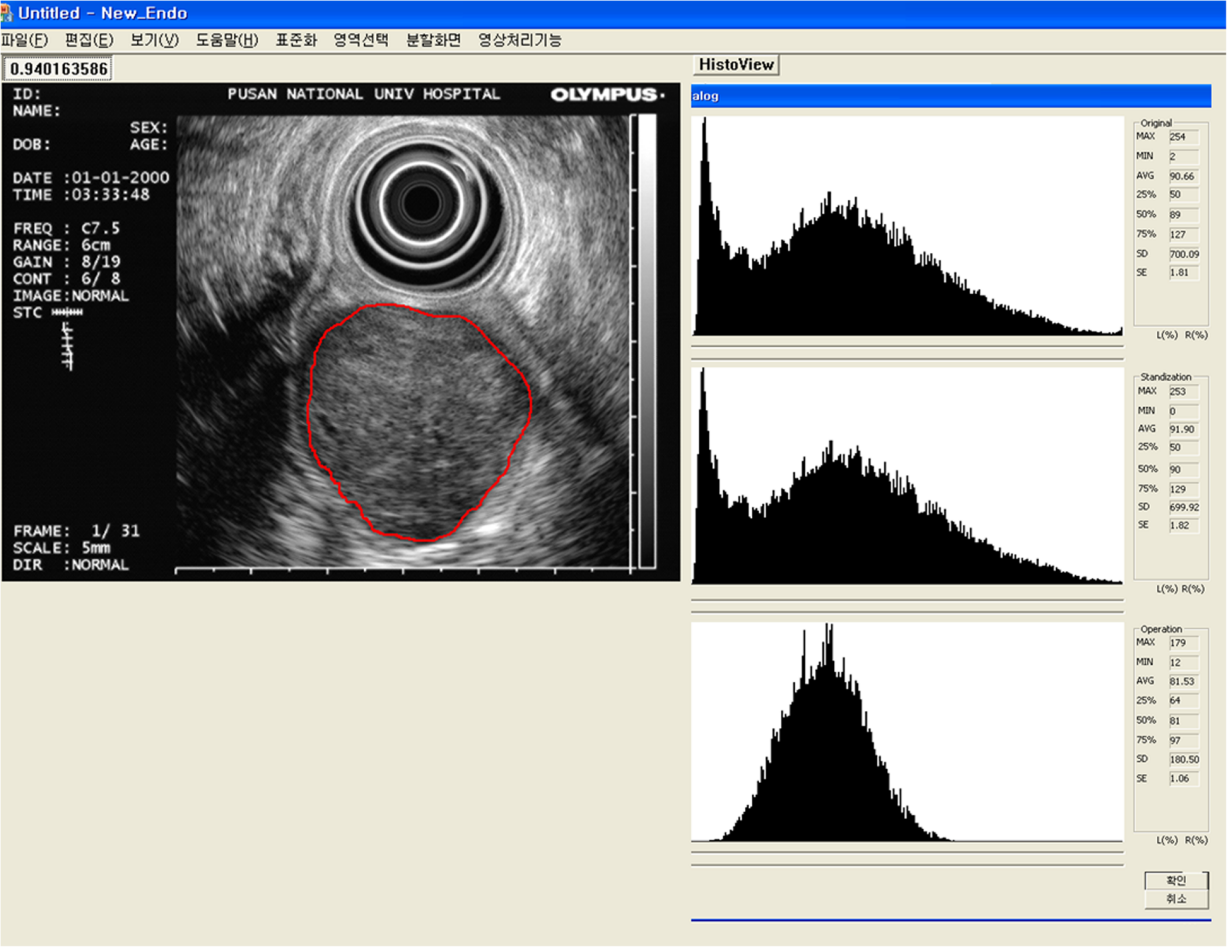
An example of digital image analysis. From the standardized image, a region of interest (ROI) is selected by an experienced endosonographer for tumor analysis. The final results for the ROI are expressed in the bottom histogram. The mean (T_mean_) and standard deviation (T_SD_) of the brightness values are 81.53 and 180.50, respectively.
